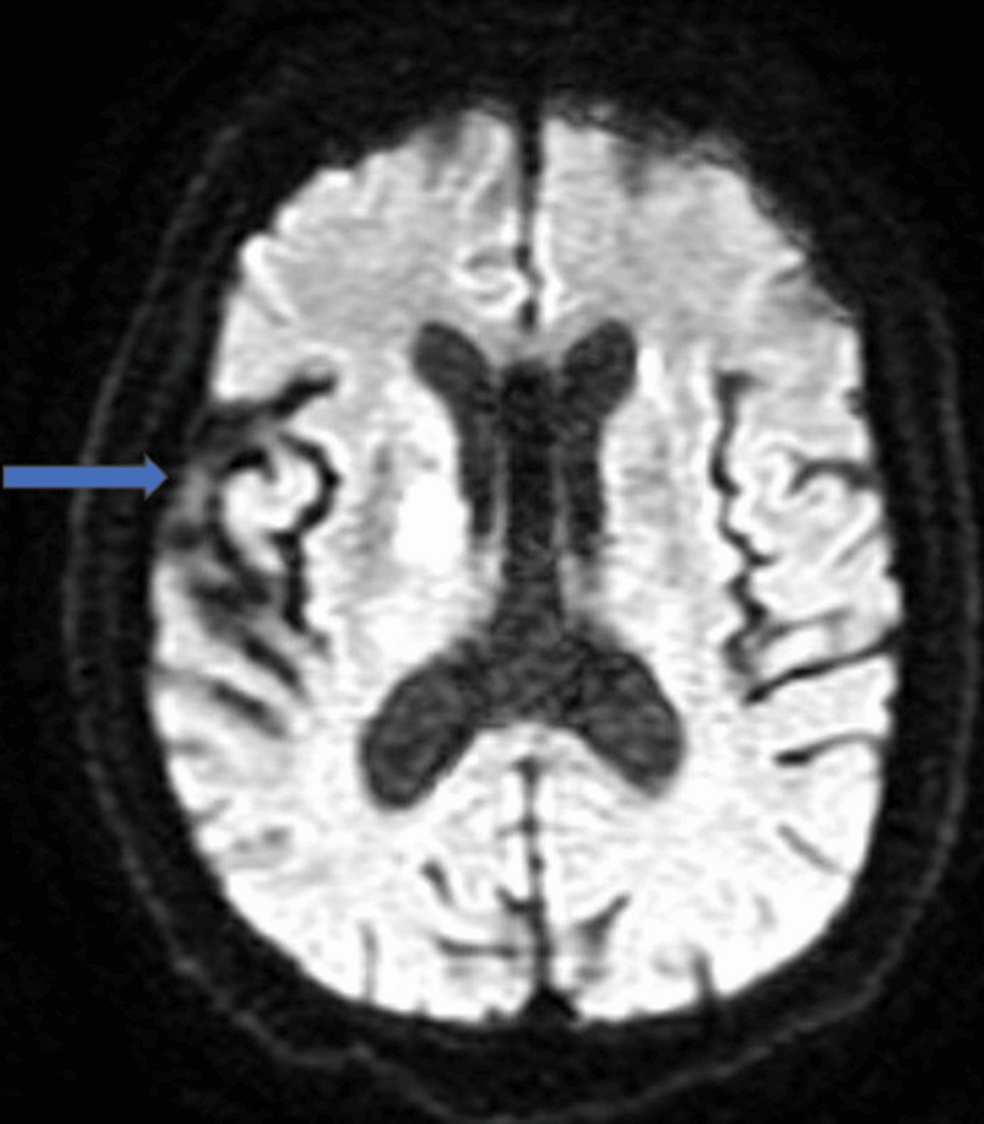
MRI brain showing infarct (blue arrow)
